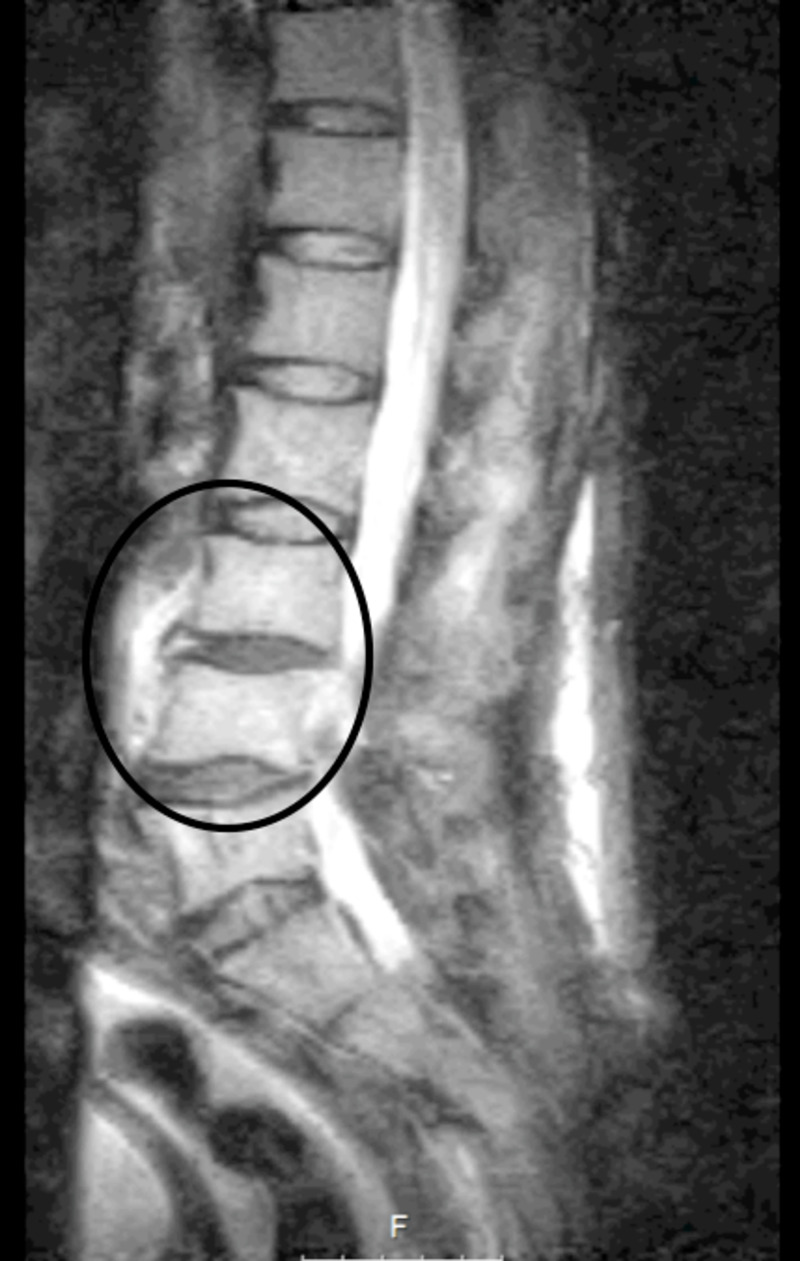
MRI T2 sequences showing L3-L4 spondylodiscitis (black circle). MRI, magnetic resonance imaging
